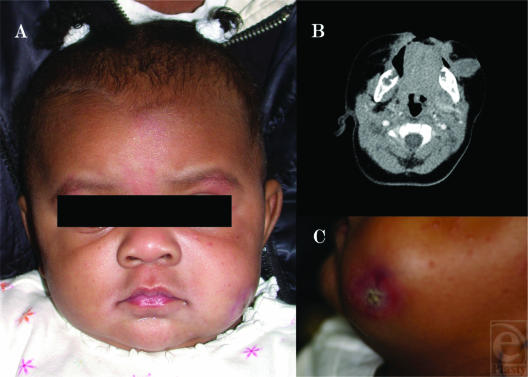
(A) The child with a rapidly growing tumor in her left cheek, shown as fullness when compared with the right cheek. (B) Computed tomographic scan showed the mass infiltrating the full thickness of the cheek. (C) Proliferating tumor with skin erosion in the overlying skin.
